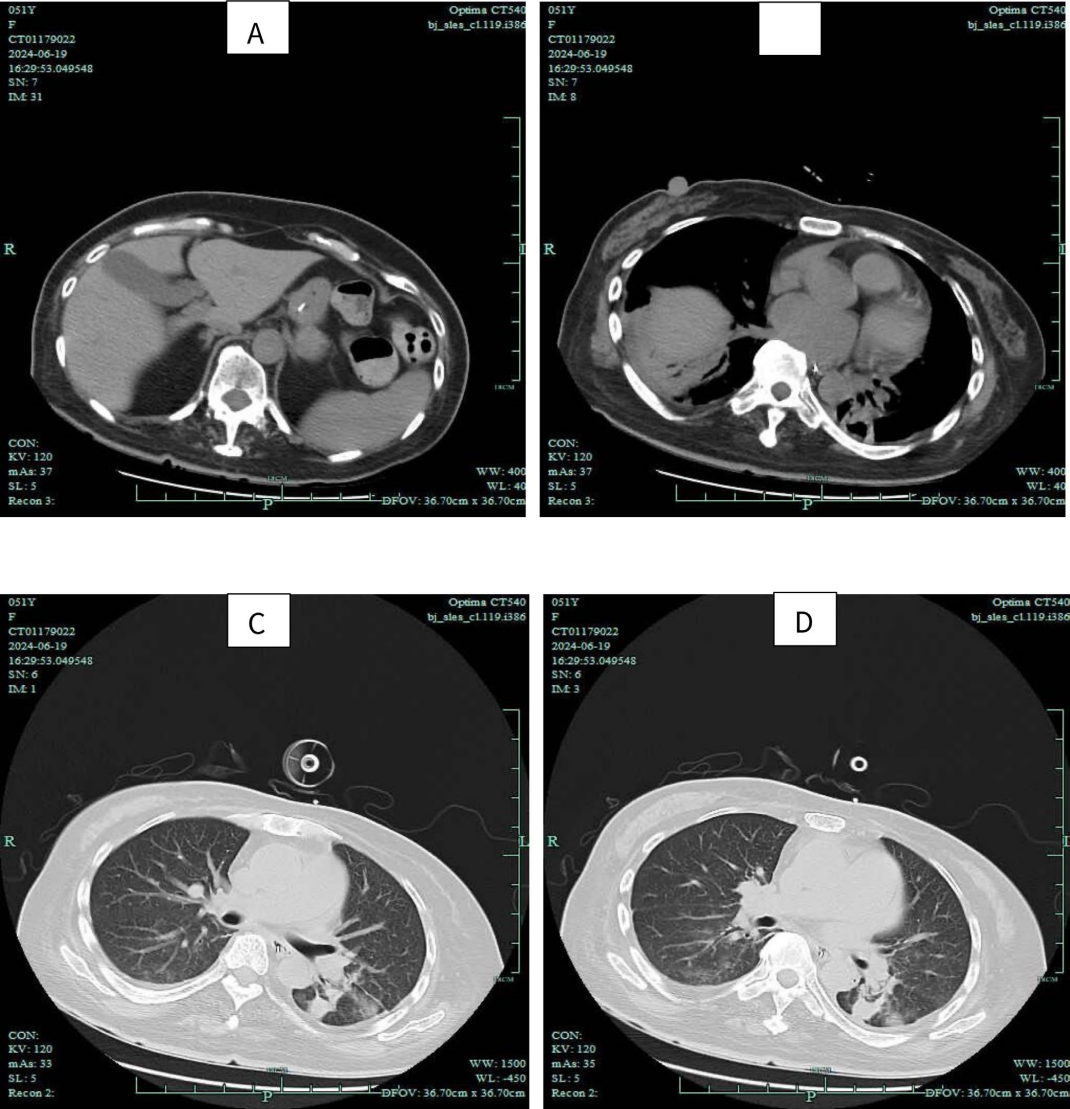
Results of noncontrast chest CT scan.
